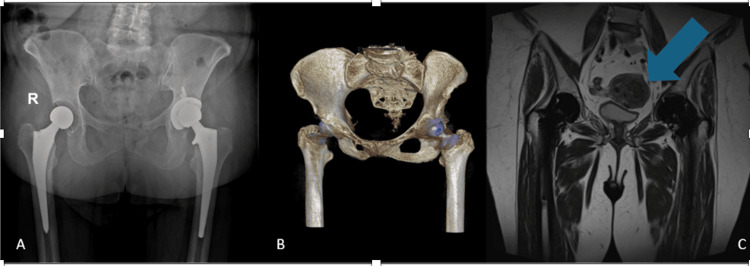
(A) Postoperative anteroposterior pelvic radiograph. (B) Pelvic three-dimensional CT scan. (C) Pelvic MRI. The arrow reveals a large leiomyoma in proximity to the left hip.
